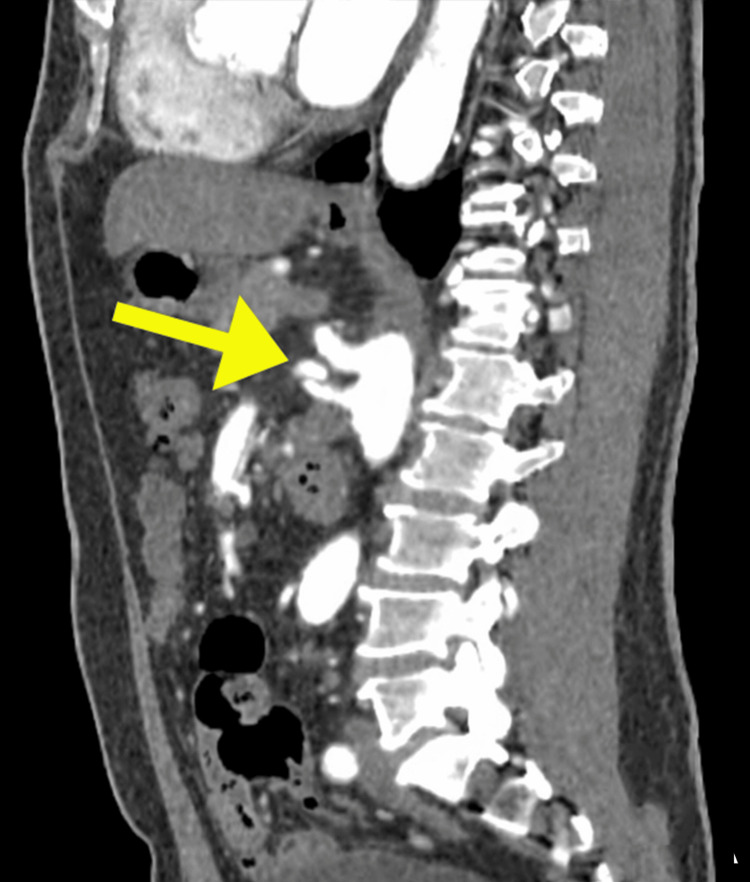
Sagittal reformatted CT image of the SMA. The dissecting intimal flap (arrow) demonstrates a dual-lumen configuration with partial thrombosis of the false lumen along the proximal SMA. CT, computed tomography; SMA, superior mesenteric artery
